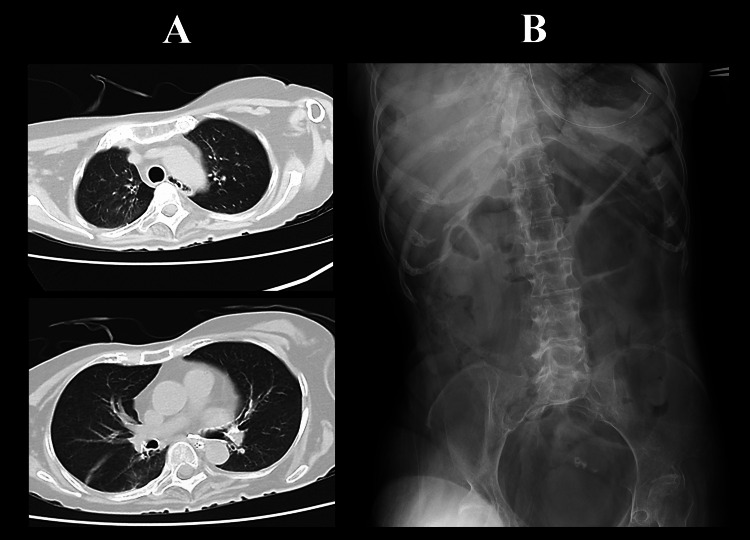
Chest CT and abdominal X-ray at discharge (A) Chest CT taken on Day 78 shows resolution of pneumonia, and (B) abdominal X-ray on the same day demonstrates no recurrence of gas retention in the stomach or intestine. CT, Computed tomography
